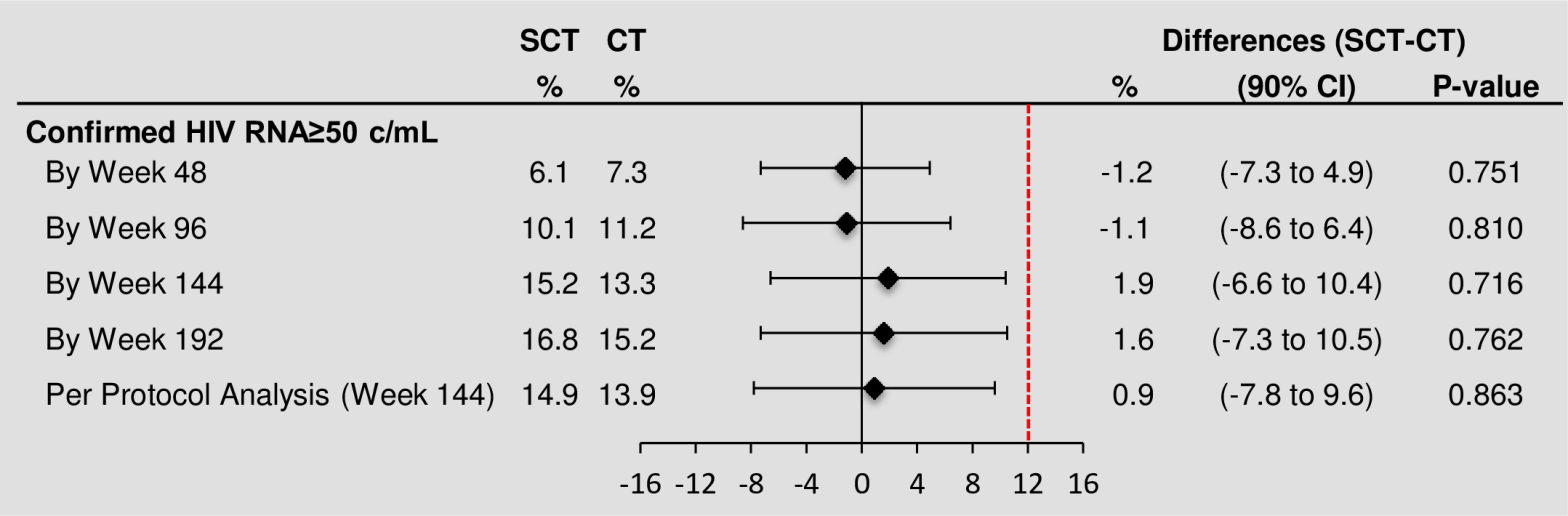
Confirmed HIV-1 RNA ≥ 50 copies/mL by 48, 96, 144 and 192 weeks. SCT = short cycle therapy, CT = continuous therapy.
